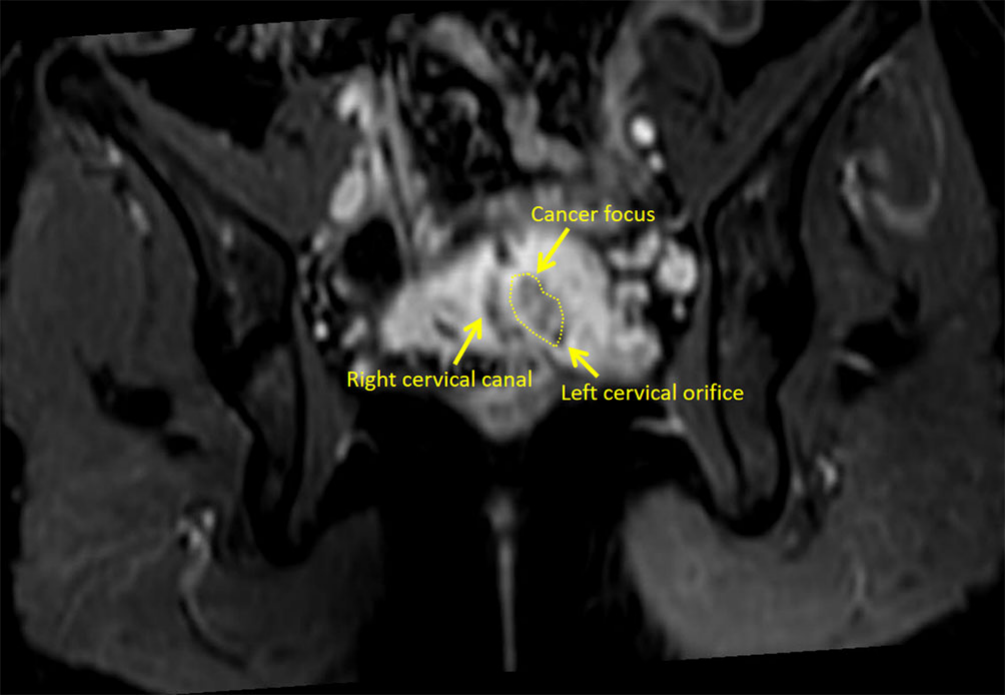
The right cervical canal and the left cervical orifice can be seen in the MRI image at this plane.
